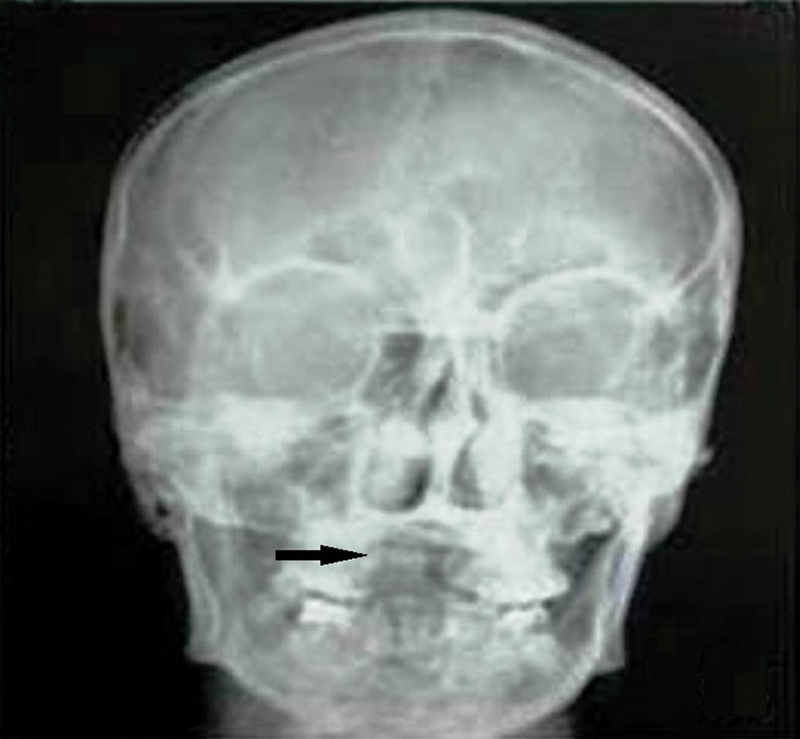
Skull X-ray demonstrating absence of upper incisor teeth (arrow).
